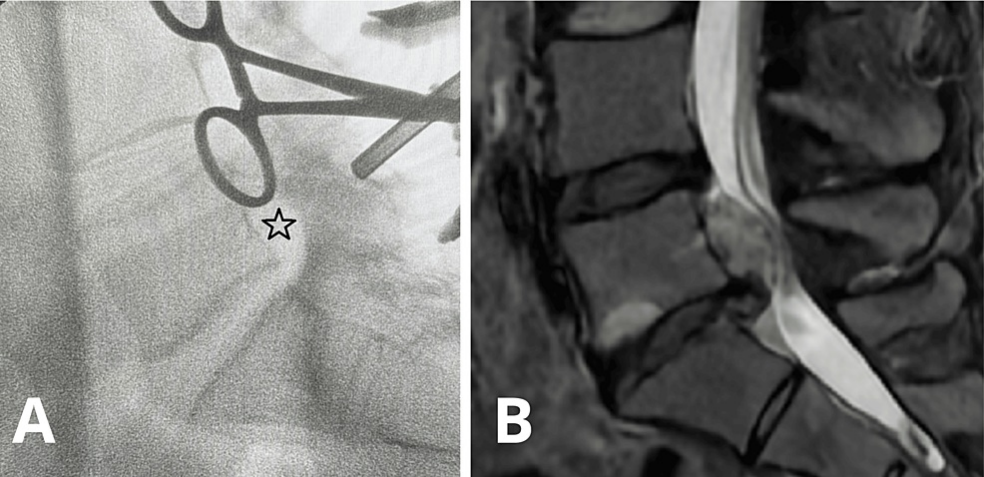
A) Intraoperative fluoroscopic image of L4-S1 levels showing the correspondence of the Caspar retractor system positioning to the preoperative MRI image (the projection of the herniated disc is marked with a black star sign). B) Sagittal view of the L5-S1 LDH on the preoperative MRI.
